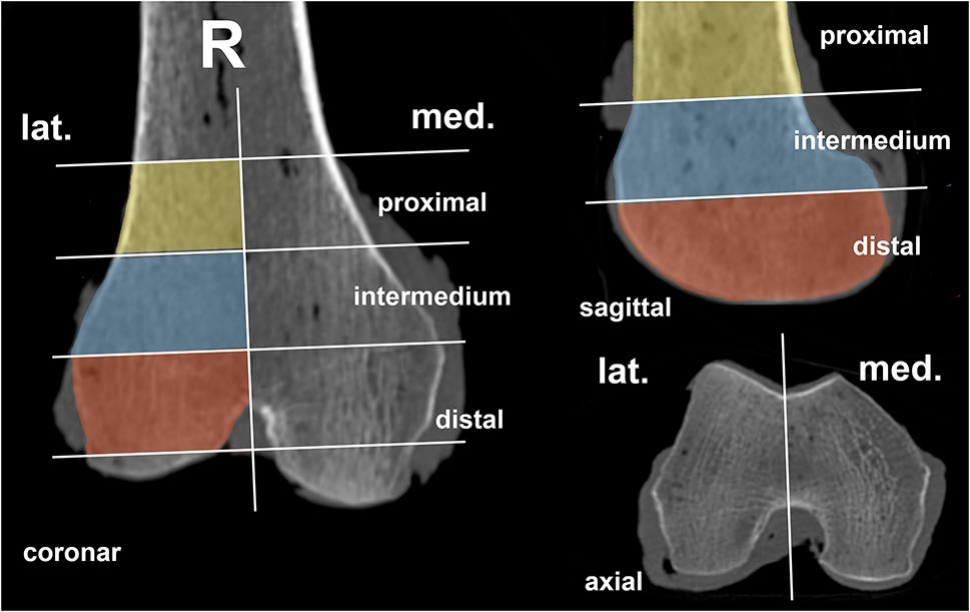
Slices of a CT scan of a right femur in the coronal, sagittal, and axial planes. The six different regions of interest (ROI) for local BMD evaluation via HR-pQCT are marked in the different planes
